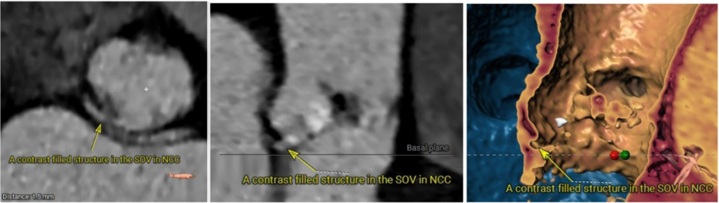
CT Representation of a Contrast-Filled Structure in the SOV of NCC A contrast-filled structure was noted in the SOV of NCC in the CT. Etiology of the finding is unknown and it could be related to prior balloon aortic valve angioplasty. Abbreviations as in Figure 1.
